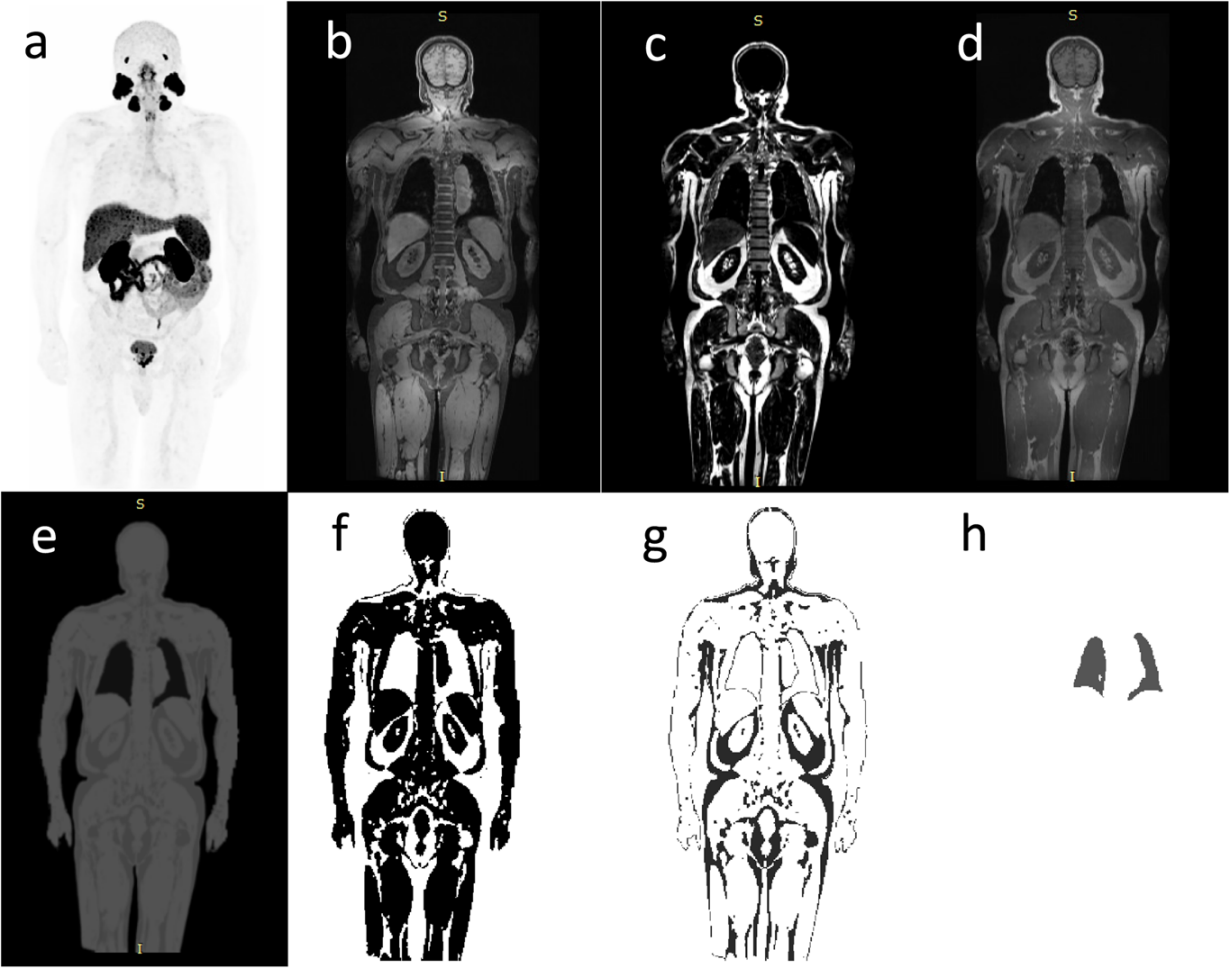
Representative images from a ^18^F-PSMA PET/MR scan. a PET MIP, b water image, c fat image, d in-phase image, e MRAC map, f water segmented from MRAC, g fat segmented from MRAC, and h lung segmented from MRAC
